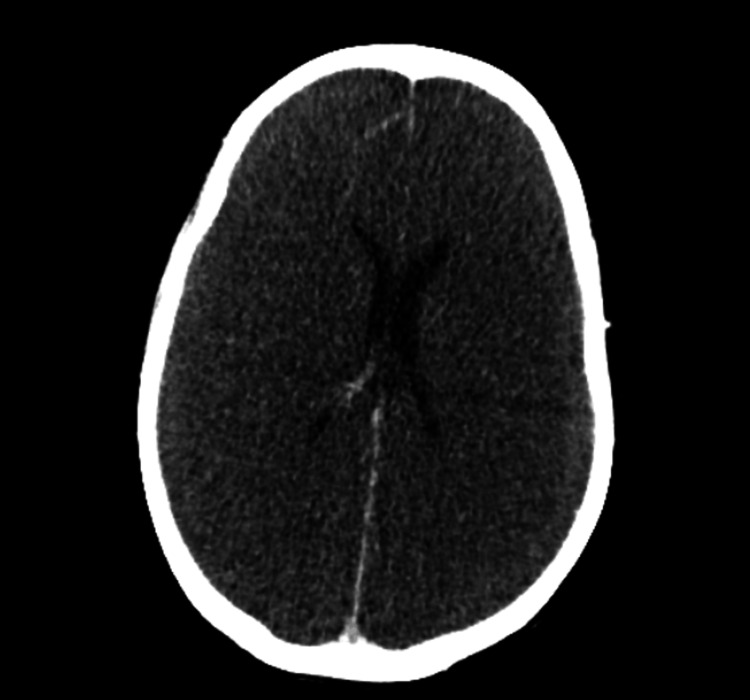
CT head study demonstrating diffuse cerebral oedema, loss of grey-white differentiation, and ventricular compression
